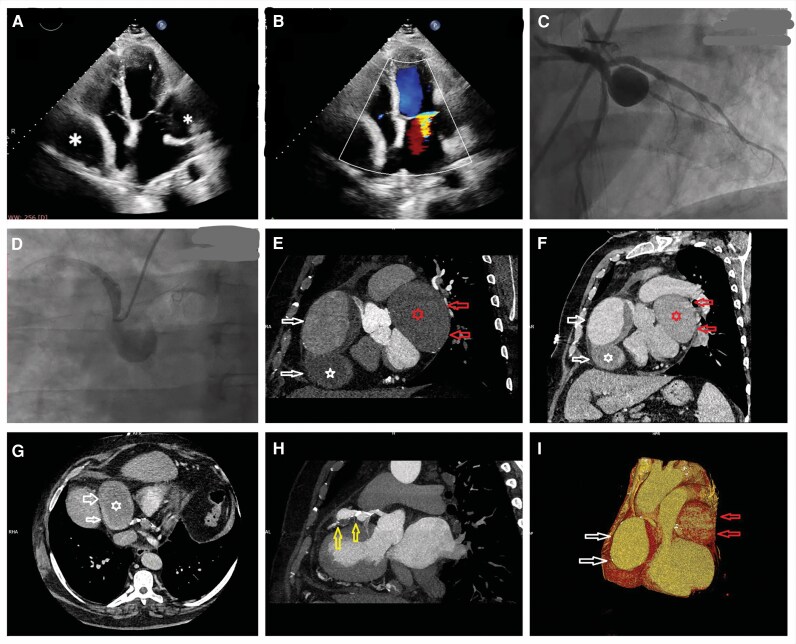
Echo-free masses (asterisks) adjacent to the left and the right atrium (*A* and *B*). Coronary angiography revealed three-vessel disease and aneurysms of the LAD, LCX (*C*) and RCA (*D*). Multidetector coronary computed tomography angiography (*E–I*) with multiplanar reconstruction of figures demonstrate the huge aneurysm of mid-to-distal portion of RCA with maximum diameter ∼80 mm (white arrows) and mural thrombosis (white stars), explaining the echo-free structure adjacent to the right atrium (*E–G*). Huge aneurysm of the proximal portion of LCX with maximum diameter ∼82 mm (red arrows) and contains thrombosis (red stars) (*E* and *F*). There are 4 different size saccular and fusiform aneurysms (maximum diameter 13.8 mm) with partial thrombosis in proximal to mid portion of LAD (yellow arrows) (*H*). The volume rendering reconstruction show aneurysms of RCA and LCX (*I*).
